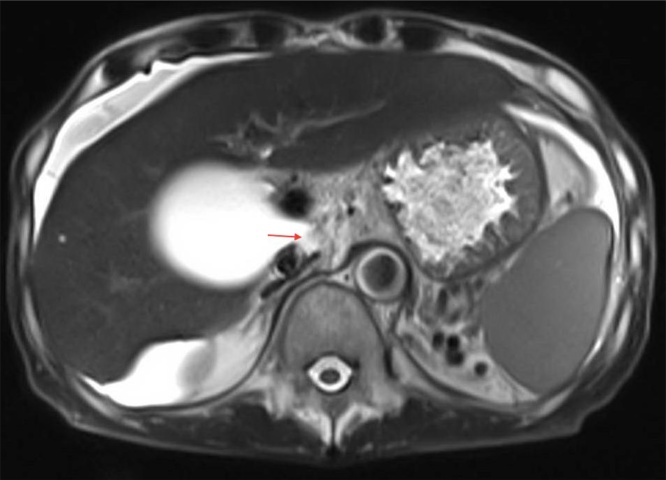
T2-weighted image demonstrating a hypointense focus in the expected locations of valves of Heister, depicted by a red arrow.
